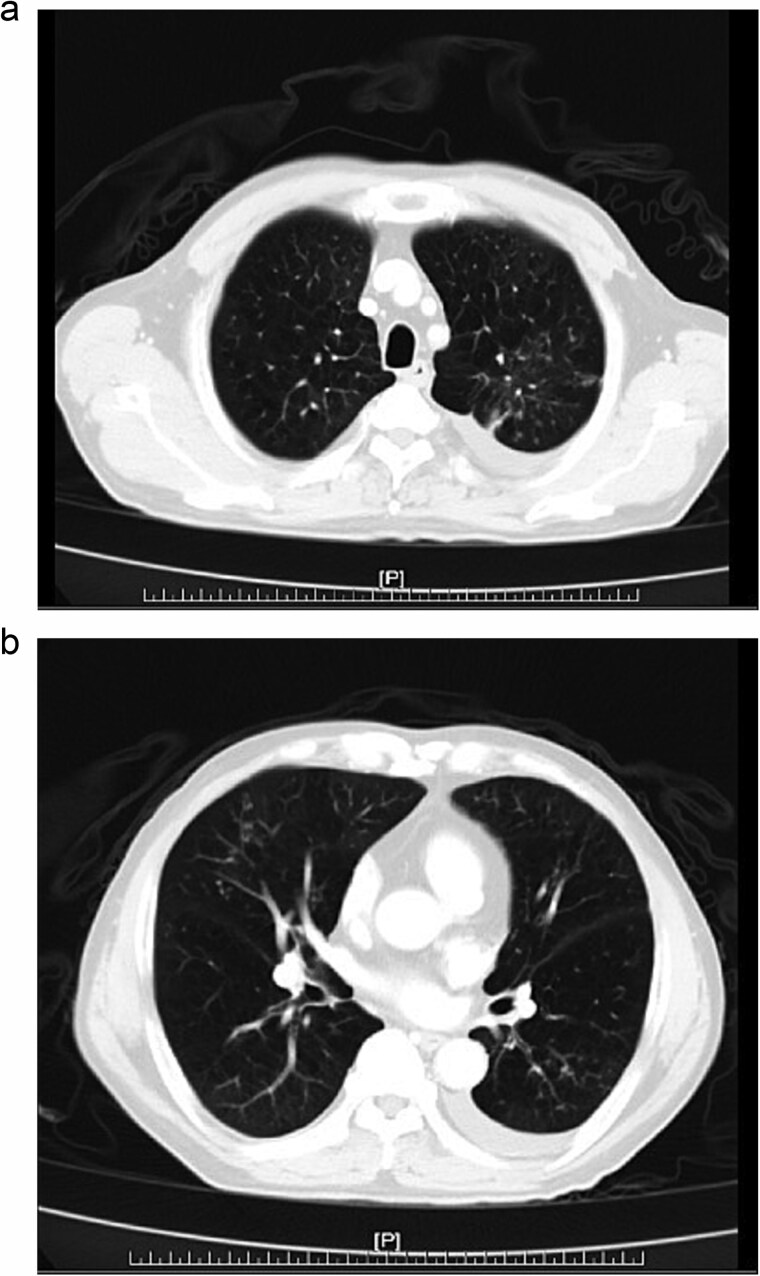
(a, b) Chest CT, multifocal of patches of centrilobular nodules and tree-in-bud in bilateral lungs.
